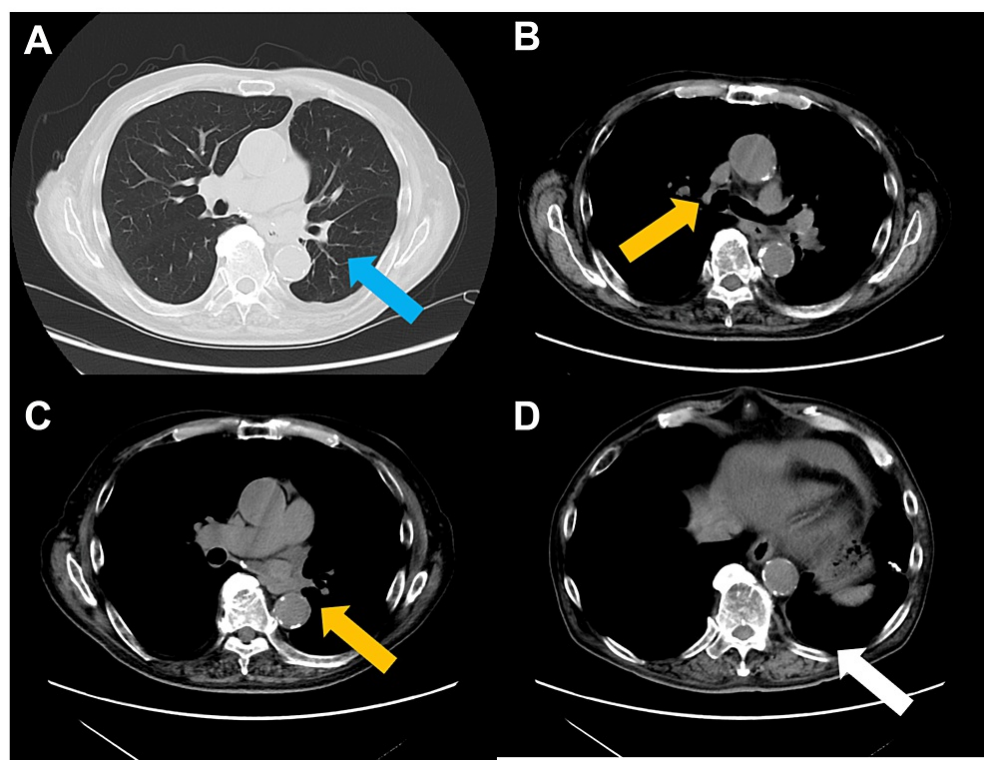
Chest computed tomography after two years from discontinuation of immune checkpoint inhibitor. Chest computed tomography demonstrated complete remission (A) primary lesion (blue arrow), (B and C) lymph nodes (yellow arrow), and (D) pleural dissemination (white arrow). A complete response was maintained.
